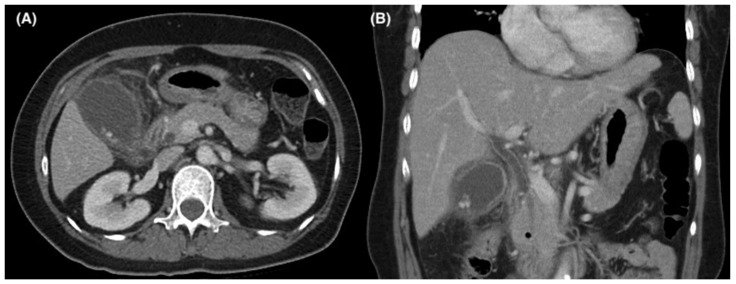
Axial (A) and coronal (B) computed tomography images of a patient with concurrent acute cholecystitis and cholangitis.
